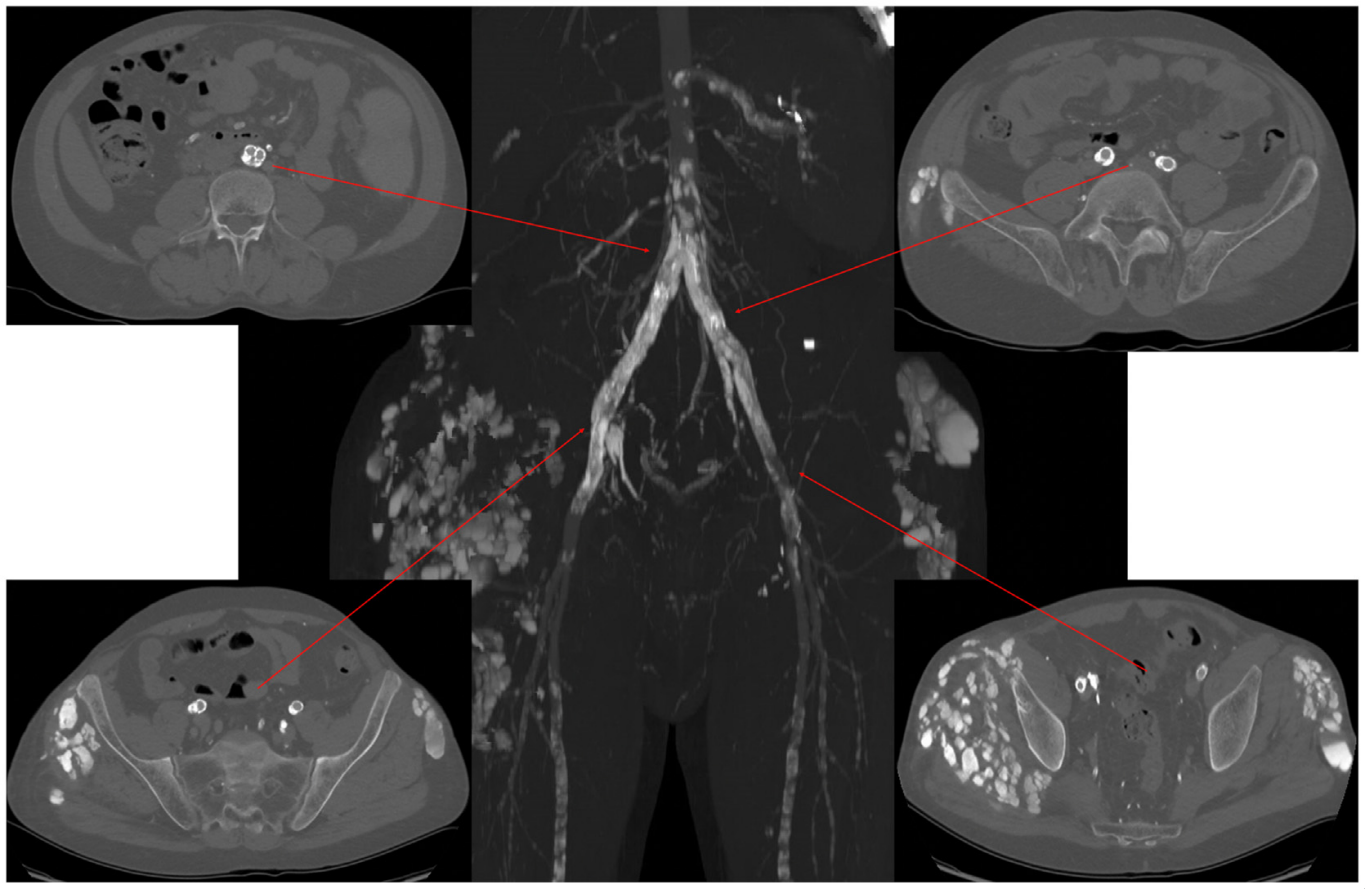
Three-month computed tomography (CT) scan.
